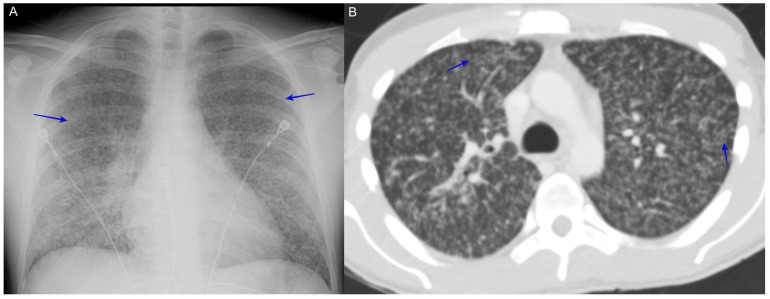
(A) A chest X-ray shows diffuse reticulonodular interstitial miliary nodules (blue arrows). (B) A CT scan shows diffuse reticulonodular interstitial miliary nodules (blue arrows). The patient was a 27-year-old man with a history of human immunodeficiency virus (HIV) non-compliant on his HIV medication who presented with a duration of cough with yellow expectoration, night sweats, chills, severe weakness, and shortness of breath upon exertion. He was diagnosed with severe coccidioidomycosis via respiratory cultures and coccidioidomycosis fungemia.
